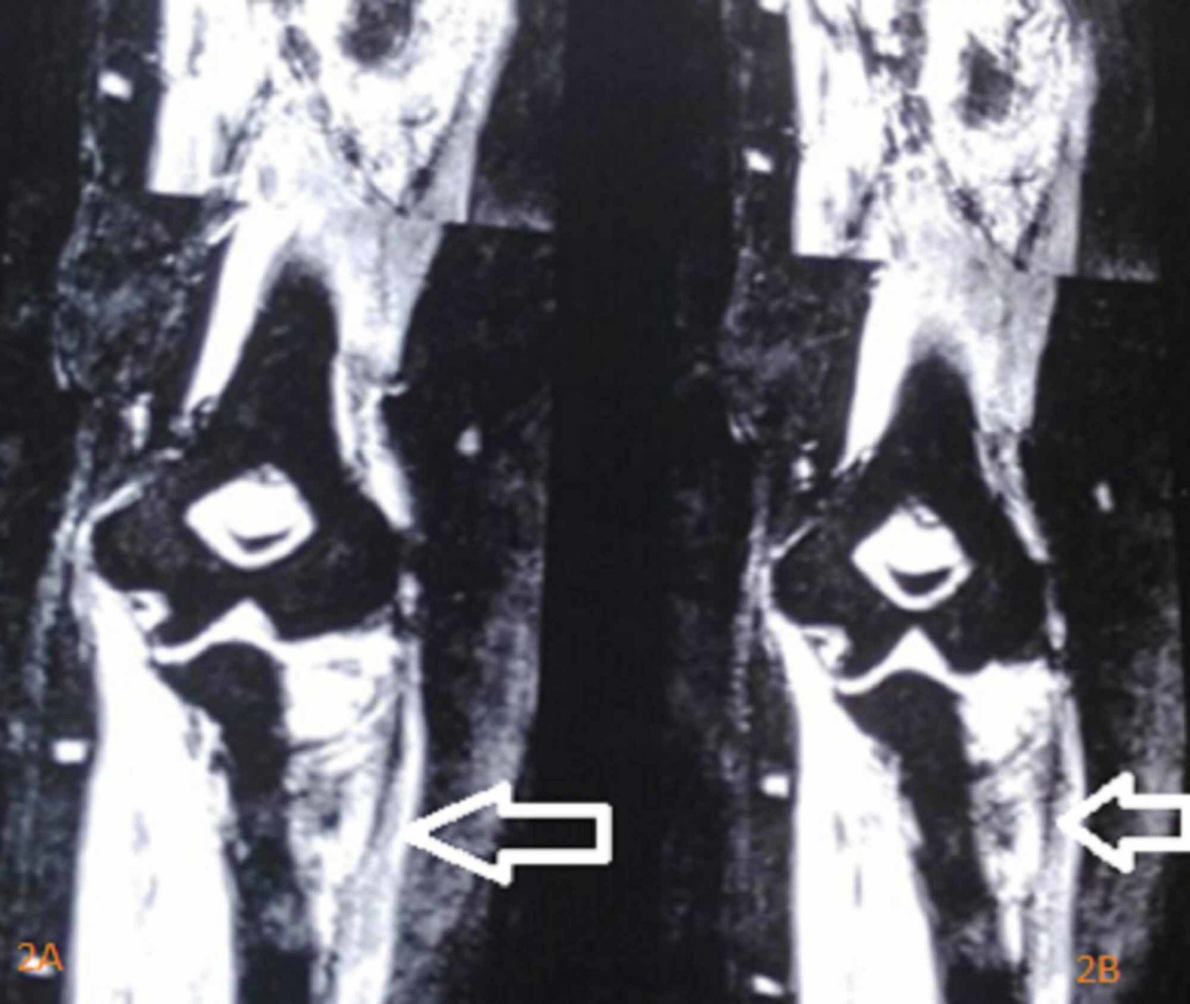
MRI scans of the left elbow MRI scans of the left elbow showing a 4.5 × 3.5 × 2 cm soft tissue lesion in the anterior part of the proximal radius with erosion of the anterior part of the head and neck of the radius. The lesion is hyperintense on T2 fat-suppressed view. Periosteal reaction is seen in the neck of the radius. Effusion and synovial thickening are suggestive of tuberculosis of the elbow.
